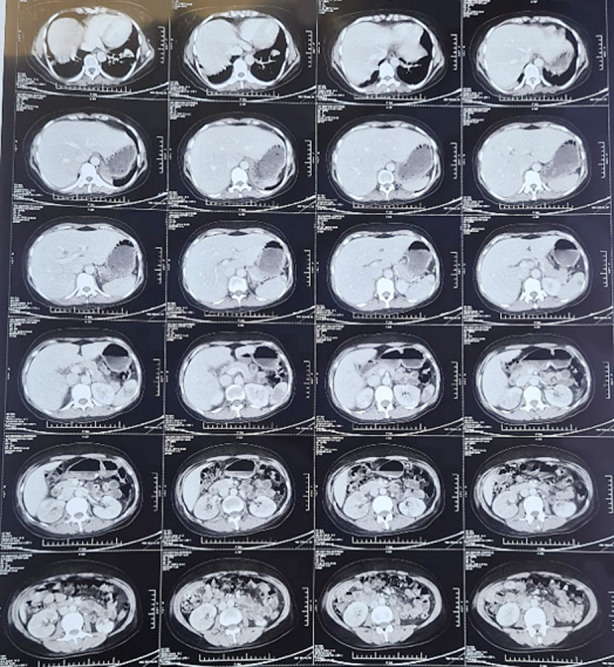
CT abdomen showing splenic and renal cortical infarcts.
